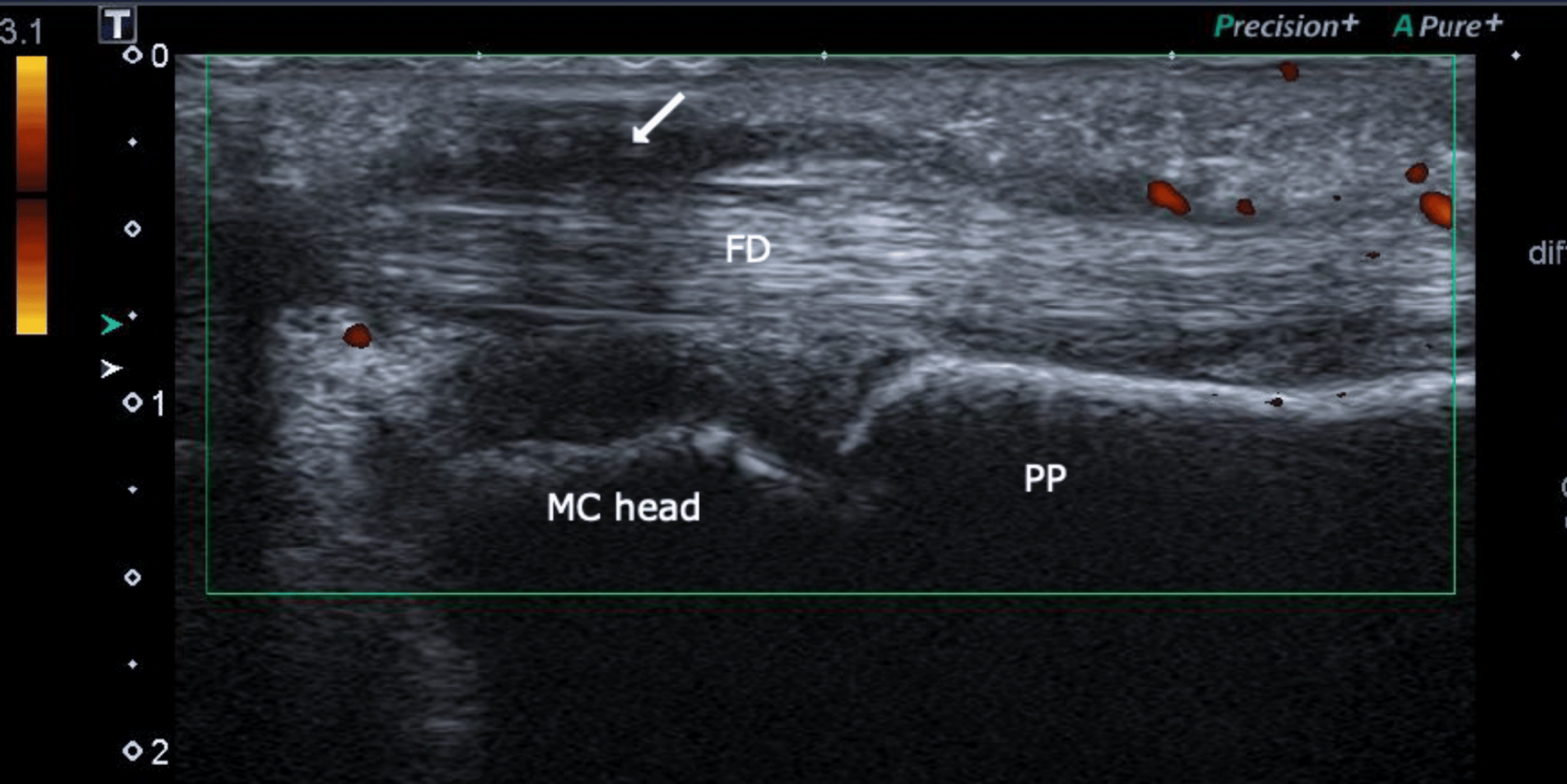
Long-axis ultrasound scan of the second flexor digitrorum of the right hand showing synovial hypertrophy (white arrow) FD: Flexor digitorum; MC: Metacarpal; PP: Proximal phalanx
